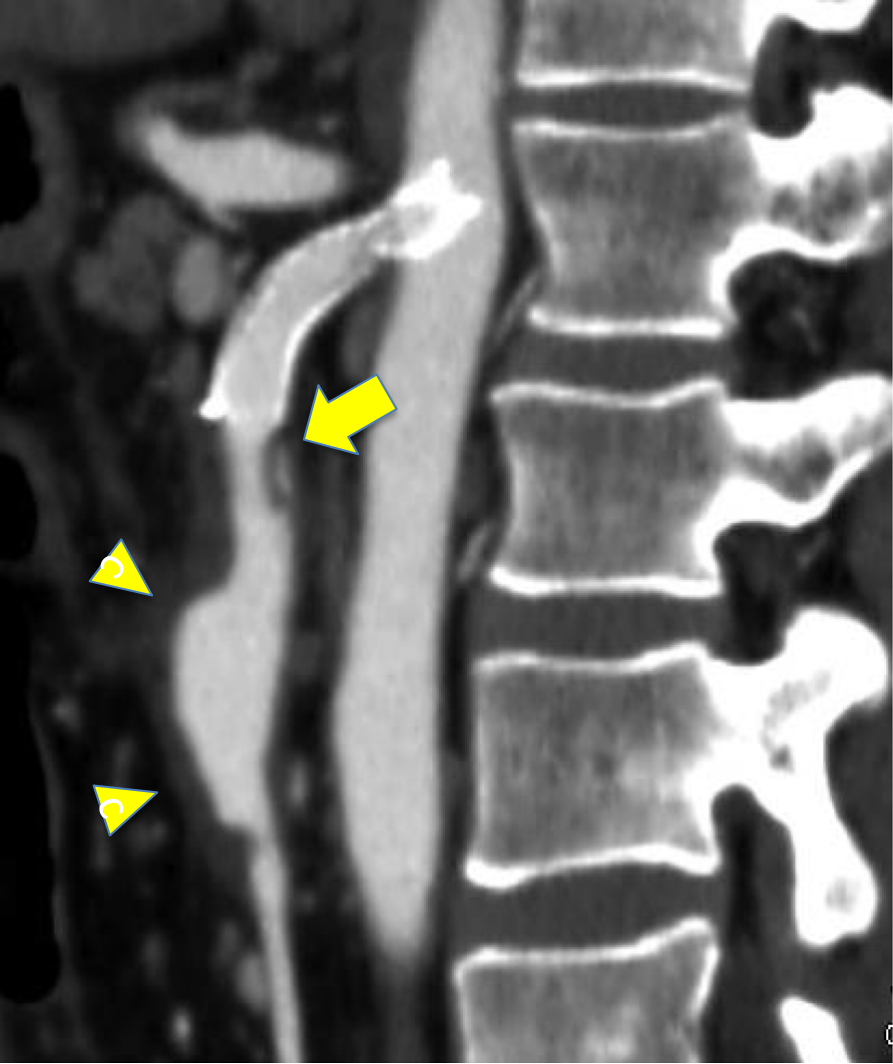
Computed tomography image at 91 days postoperatively. The true lumen of the superior mesenteric artery was dilated at the patch angioplasty site (arrowheads), the stent was patent, and a small area lacking contrast medium was present at the remaining flap (arrow)
